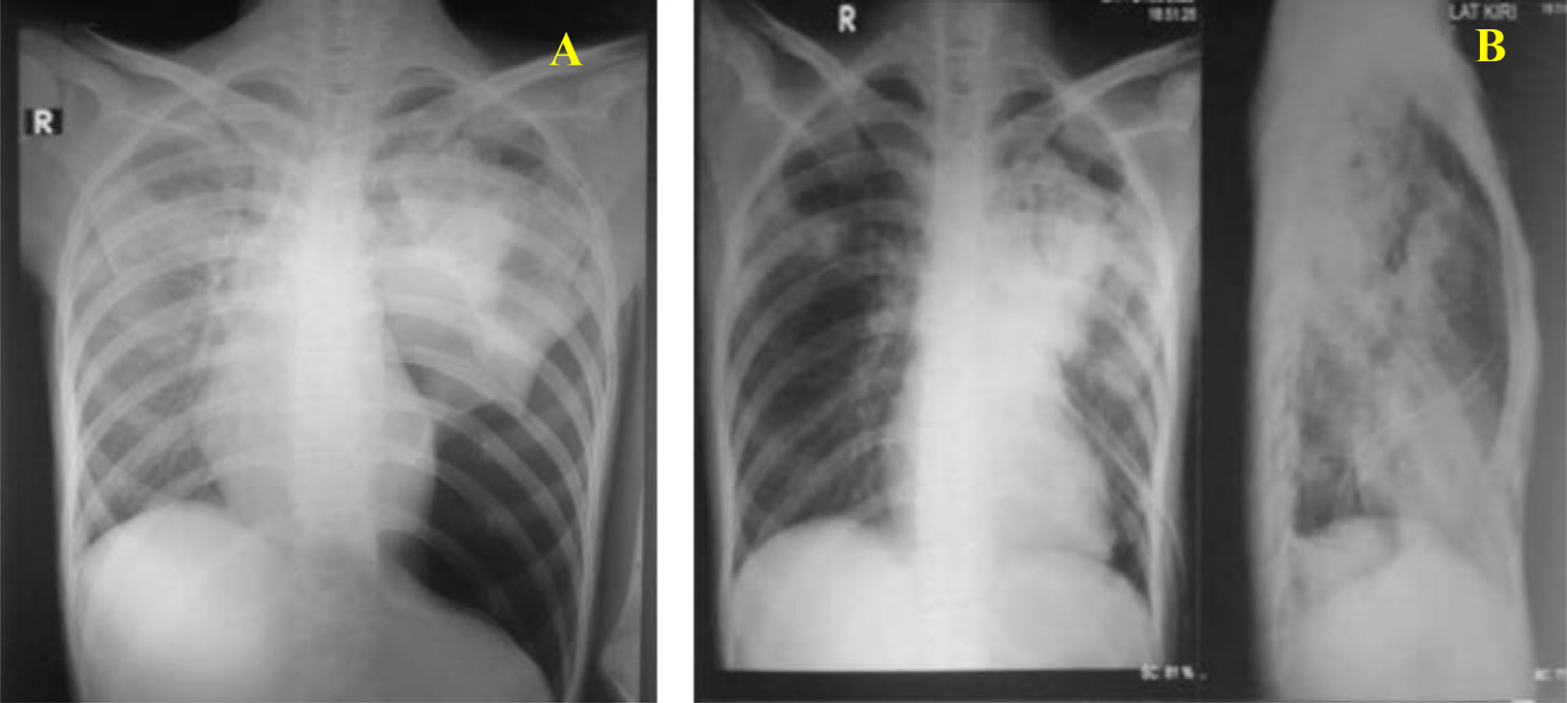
The result of AP chest X-ray of the patient on the first day of hospitalization. (A) an impression of pneumothorax was obtained and (B) a chest tube was placed.
